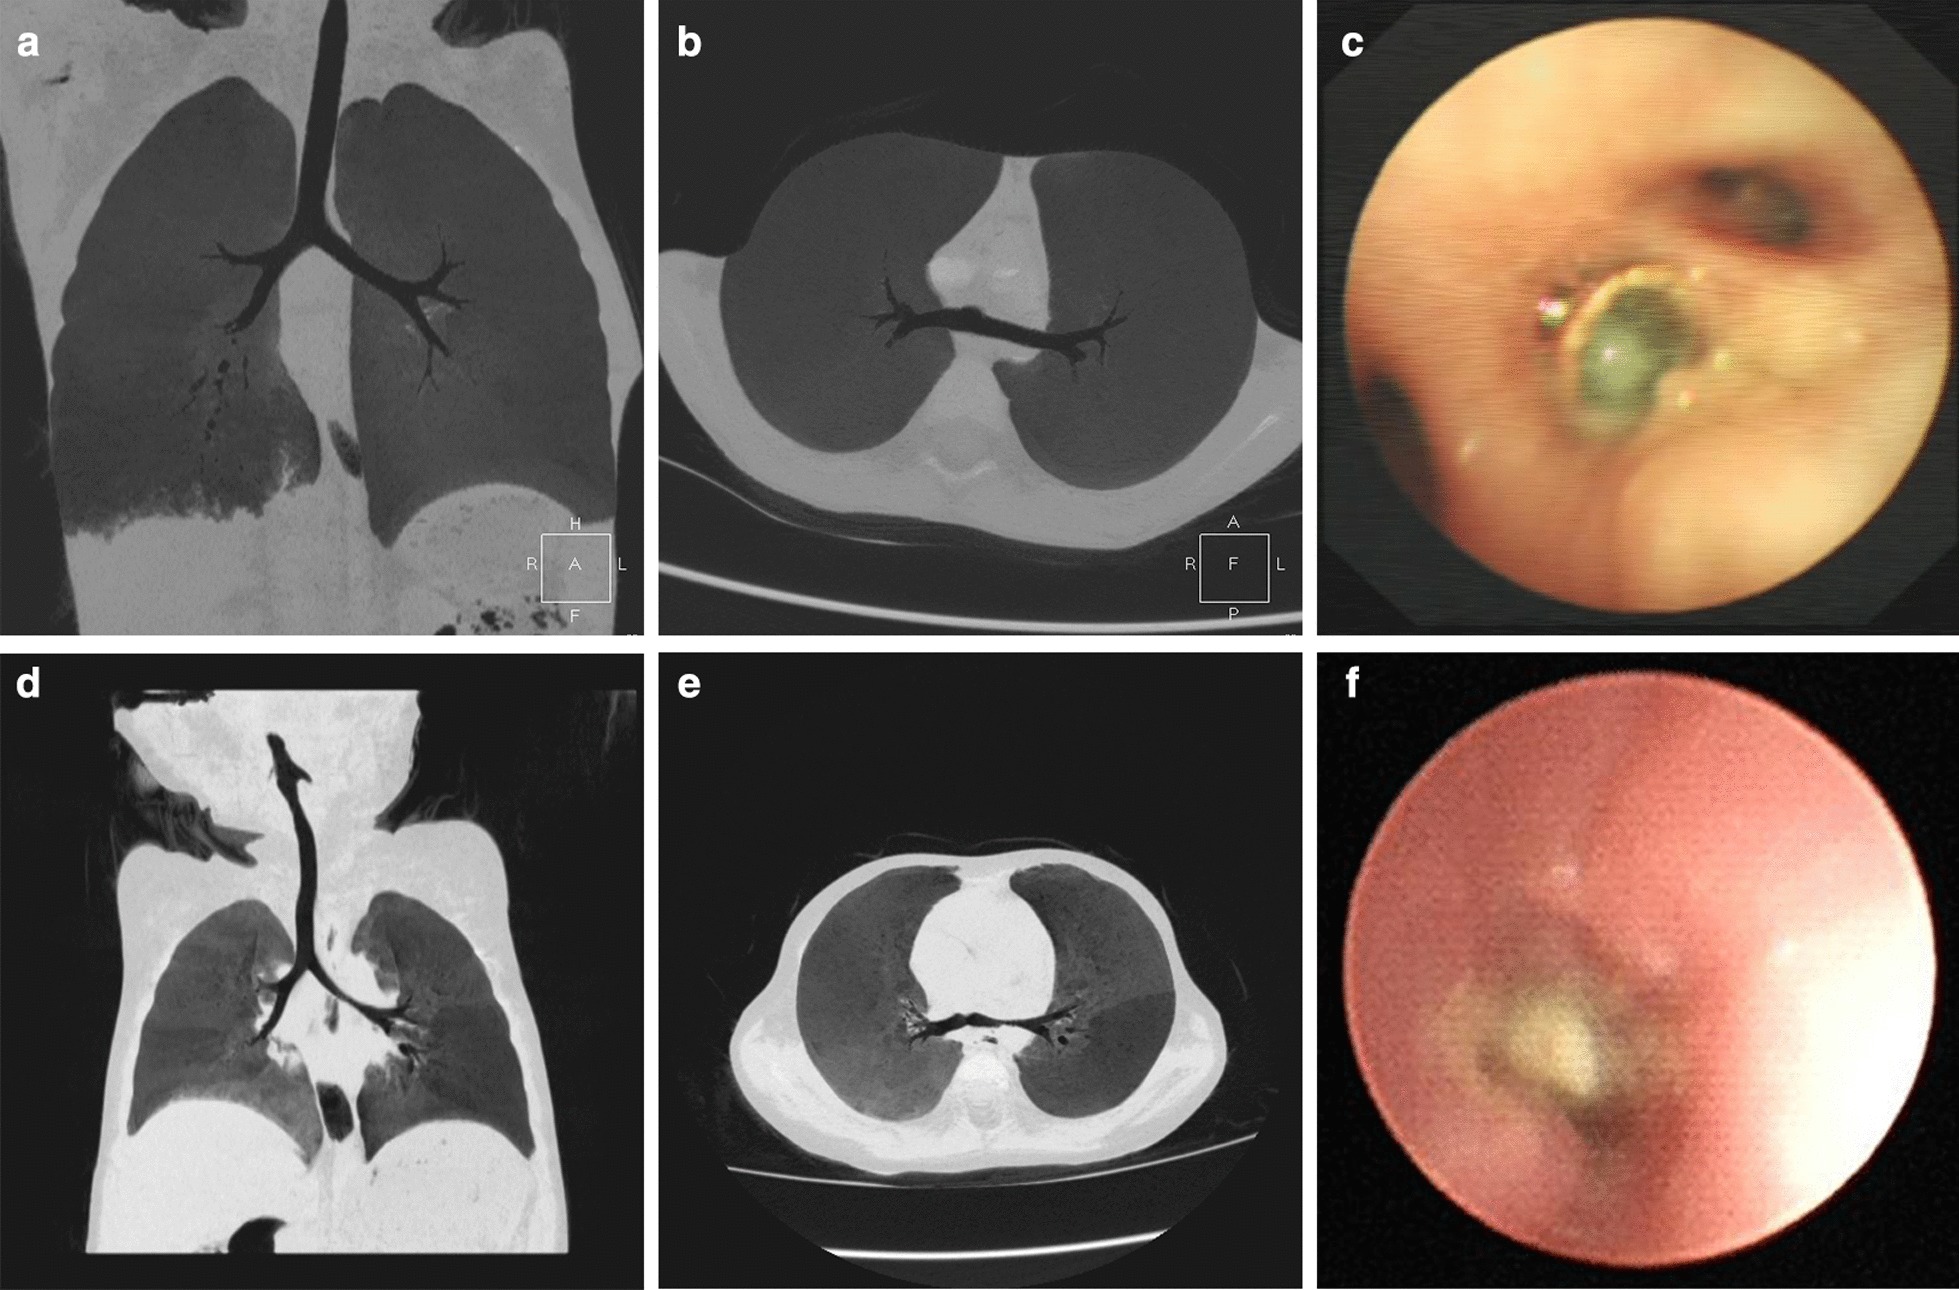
Multi-slice spiral CT (MSCT) and endoscopy of occult FBA. a-c CT showed right lower lobe lesions with bronchiectasis, and the distal bronchus was not very smooth. Clinically, sputum plugs were considered. Foreign body (red pepper) was occasionally found in the basal segment of the right lower lobe during bronchial lavage. d-f CT showed uneven inflation of the lungs and stenosis of the left main bronchus. We considered the presence of congenital pulmonary dysplasia. The symptoms did not ease after treatment. Endoscopy revealed a foreign body (peanut) in the left main bronchus
